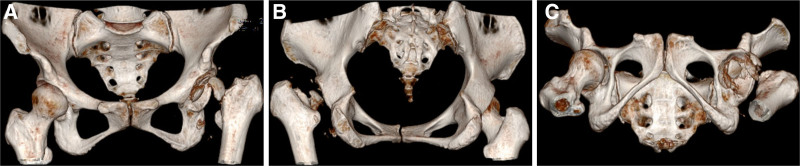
(A–C) Preoperative 3-dimensional computed tomography examination of the hip: (A) Preoperative anteroposterior 3-dimensional computed tomography demonstrating the normal structure of the left femoral head and femoral neck disappears, and multiple irregular bone fragments can be seen, with rounded edges; the bone density of the left acetabulum decreases, and the local bone structure is irregular, and the left femur is shifted to the outside and the top. (B) Preoperative postero-anterior 3-dimensional computed tomography. (C) Preoperative bottom 3-dimensional computed tomography.
